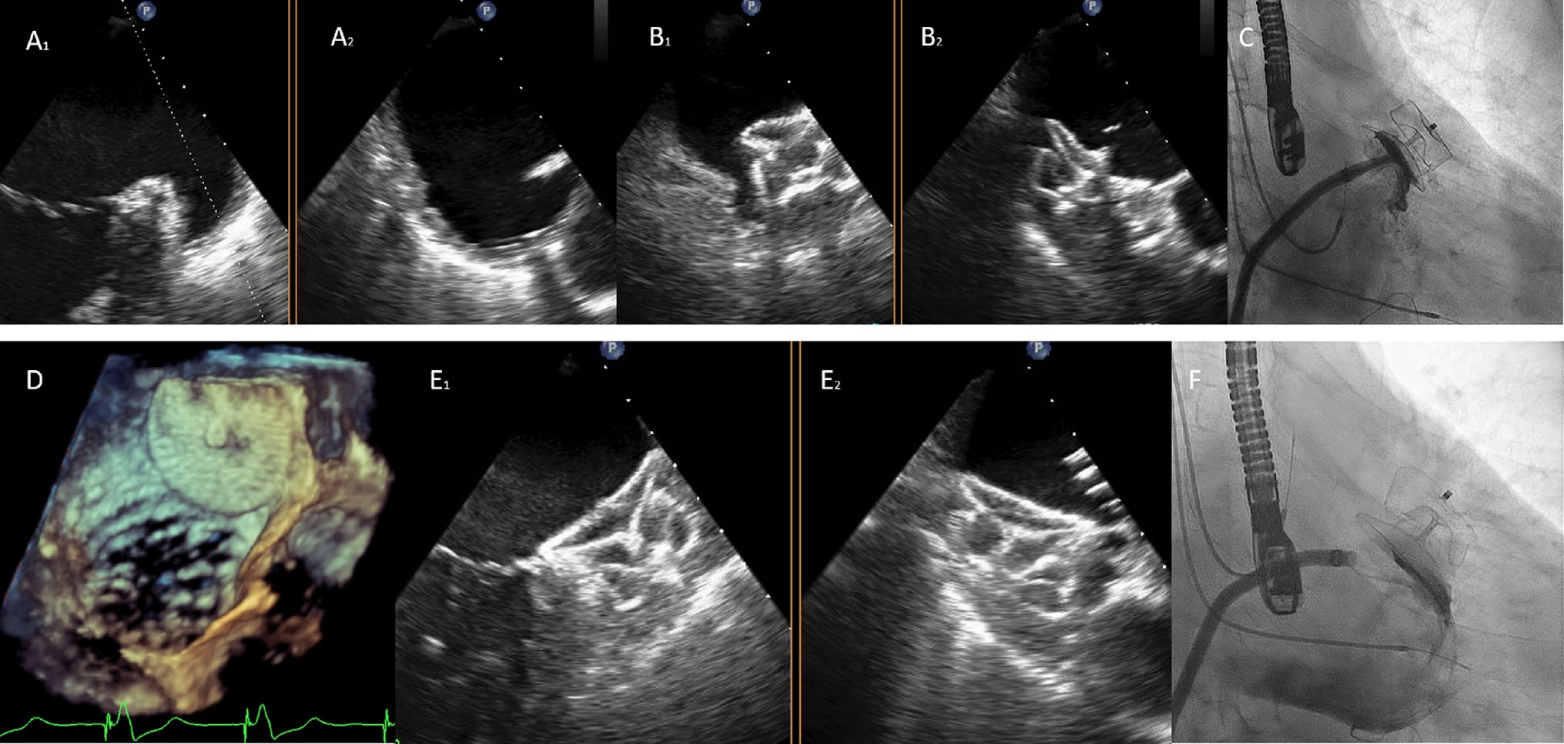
a1 and a2 Transesophageal echocardiography (TEE) of the left atrial appendage in X-plane view (65/-24). b1 and b2: the image shows a non-appropriate orientation and apposition with the 28 mm AMULET device. c Angiography showing a lack of compression on the device and although there was no contrast inside it was decided not to release the device. With the use of FEops application a 34 mm Amulet device was implanted and released after checking good apposition and compression. d tridimensional TEE image of the device. e1, e2 and f TEE and angiographic images showing correct colocation of the Amulet device
